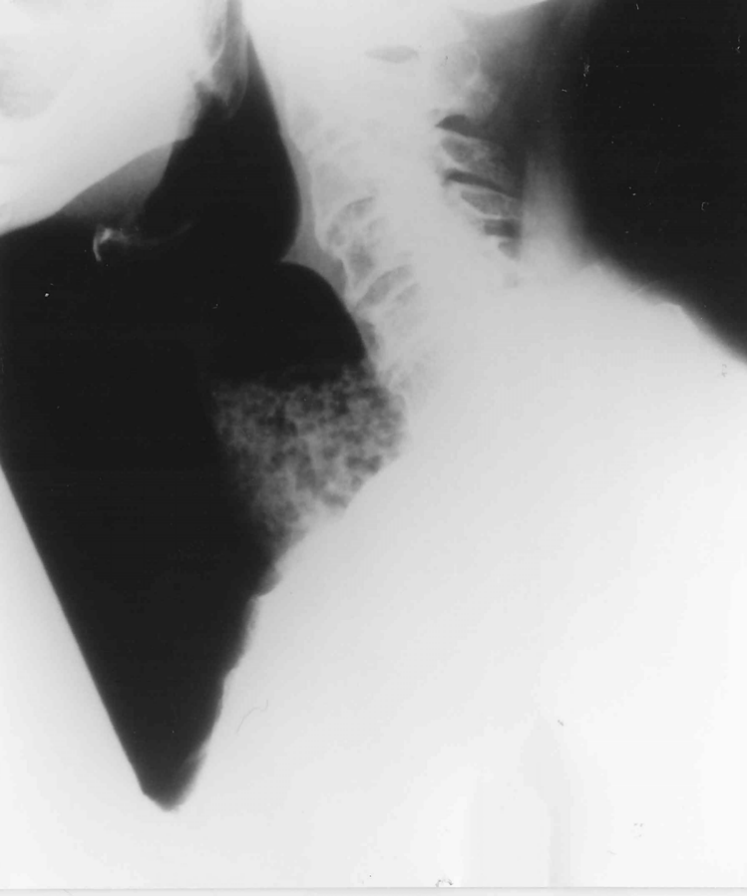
Lateral soft tissues X-ray revealing undigested material in the cervical oesophagus.
